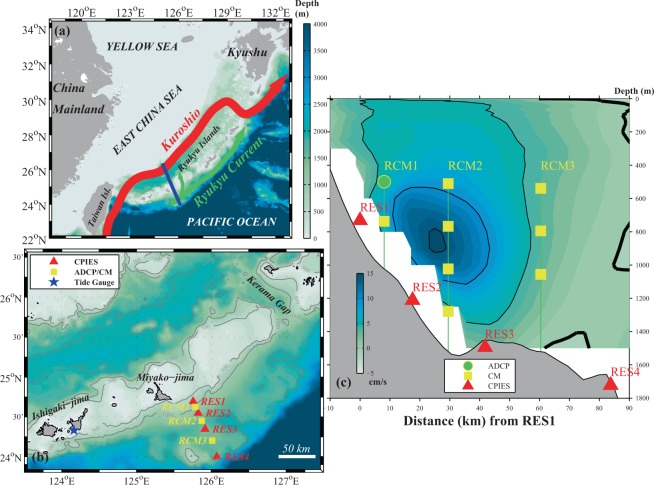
(a) Map of the East China Sea and northwestern Pacific Ocean. Kuroshio Current, Ryukyu Current (RC), and mooring section are indicated by red, green, and blue lines, respectively. (b) Mooring locations offshore of Miyakojima Island. Tall current meter (CM)/acoustic Doppler current profiler (ADCP) moorings (RCM1, RCM2, RCM3) are indicated by yellow squares; current and pressure-recording inverted echo sounder (CPIES/PIES) moorings are indicated by red triangles; the Ishigaki tide-gauge station is indicated by a blue star. (c) Cross-section of the mooring array that observed the RC. Background colour shows the long-term (Jan. 2000 to Jun. 2017) mean velocity (cm s^-1^) across the section, with black contours at intervals of 5 cm s^−1^, from the HYbrid Coordinate Ocean Model reanalysis. Bold black contour lines indicate zero velocity.
